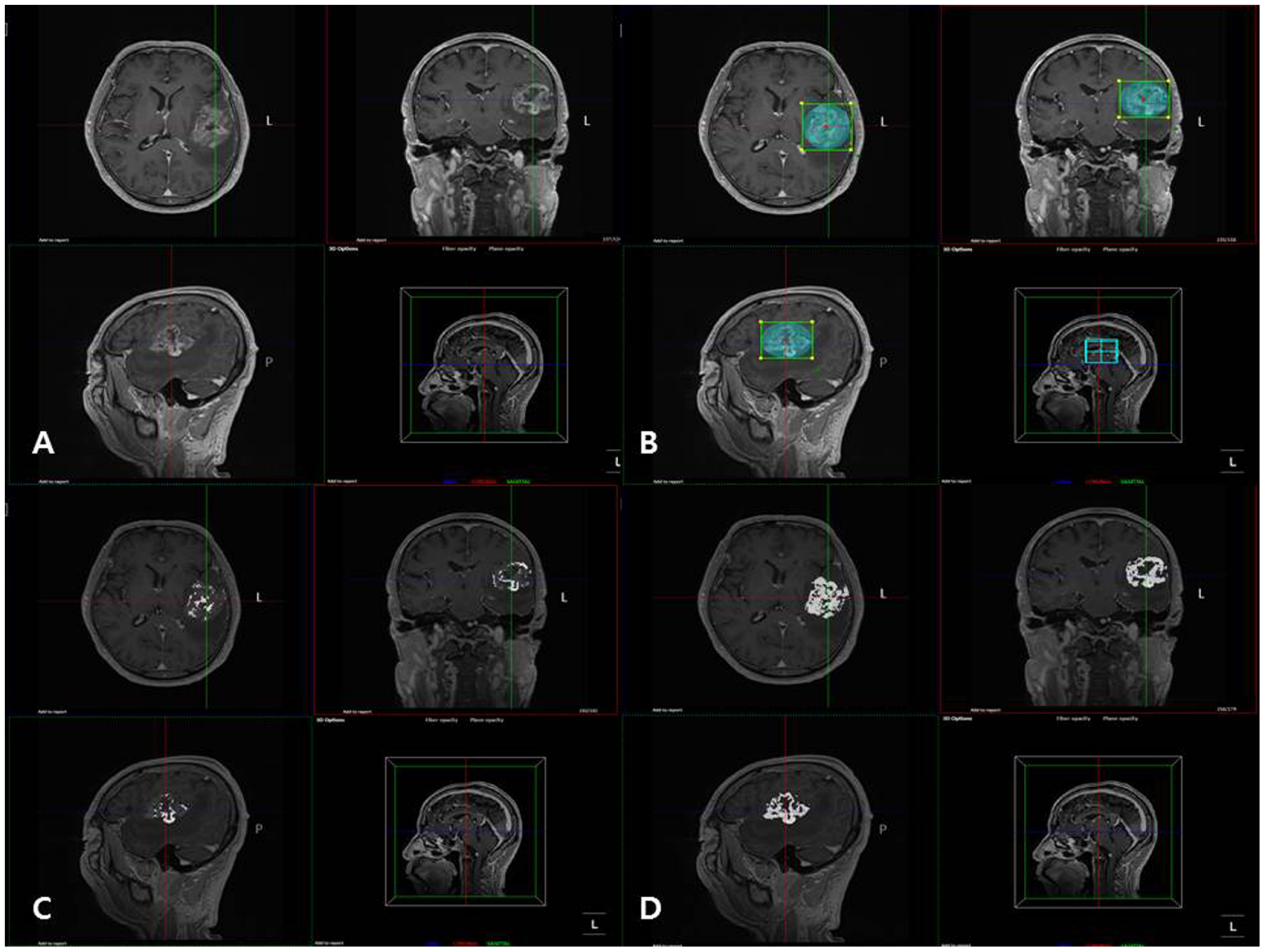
Clustering method was used as a semi-automatic segmentation of glioblastoma. (A) Contrast-enhanced T1-weighted images were used as structural images. (B) Volume of interest (VOI) was determined by a neuroradiologist. (C, D) VOIs were divided into seven tissue classes according to pixel values and class 6 and 7 were selected as enhancing tumor tissue. (C: class 6, D: class 6 and 7 together).
